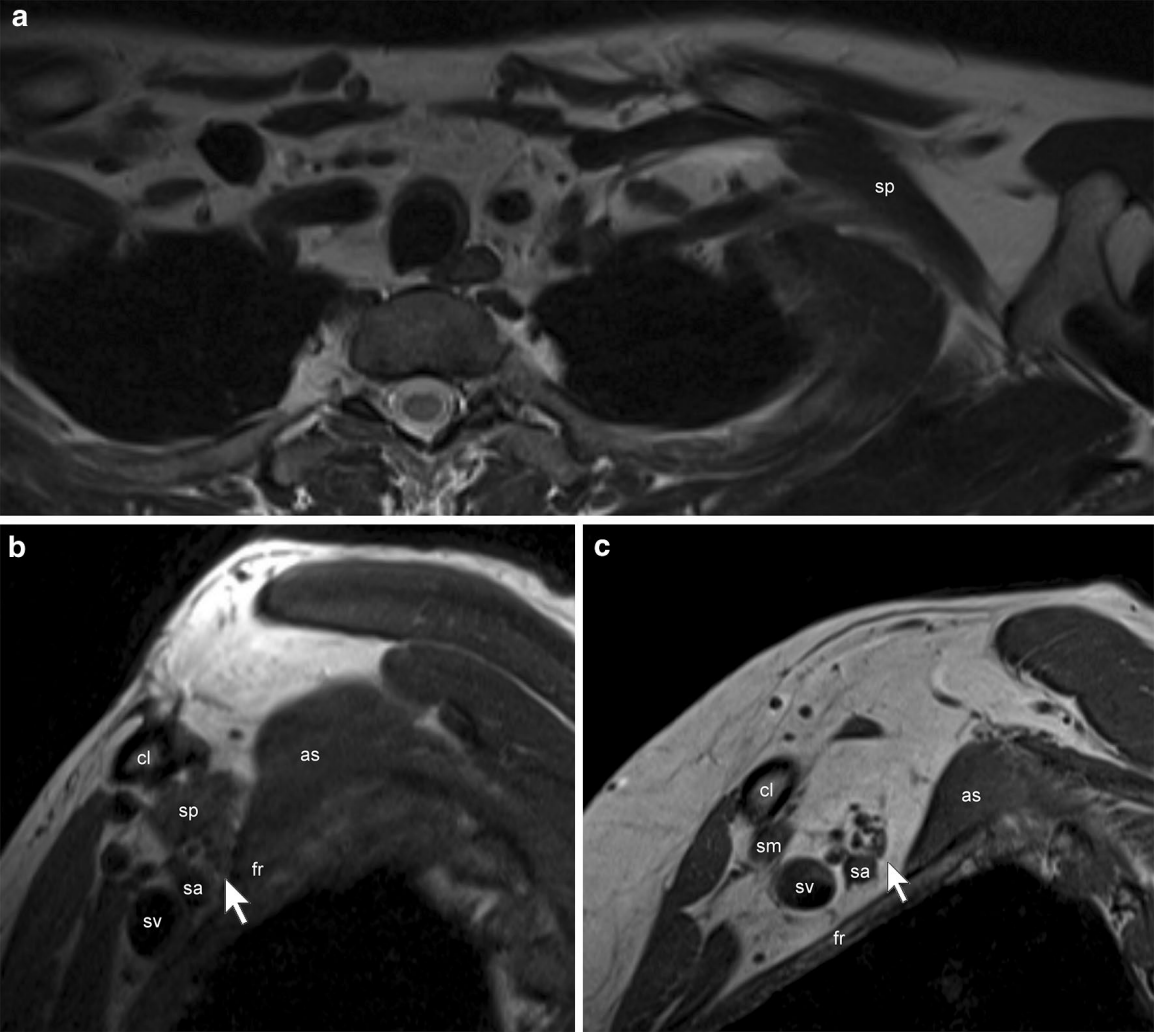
MRI of the brachial plexus at 3T (Magnetom Verio, Siemens, Erlangen, Germany), the arm adducted: a axial T2w images showed the accessory subclavius posticus muscle (*sp*) on the left side, attached to the superior border of the scapula and the medial aspect of the first rib (*fr*). No regular subclavius muscle was found. b Sagittal T1w images showed the subclavius posticus muscle coursing over the brachial plexus (*white arrow*) and the subclavian artery (*sa*) and vein (*sv*), narrowing the costoclavicular space (*cl* = *clavicle*). There was only a little fat tissue left between this muscle, the neurovascular structures, the first rib and the anterior scalene muscle (*as*). No regular subclavius muscle was found, c for comparison a normal appearing costoclavicular space with a regular, thin subclavius muscle (*sm*) of a control subject.
